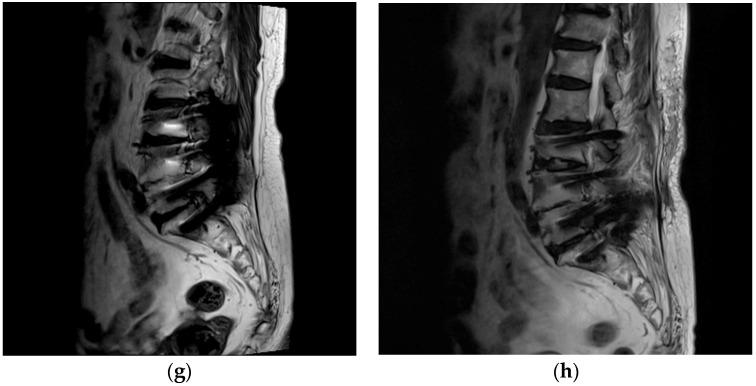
Preoperative and postoperative computed tomography and magnetic resonance images. (a) Preoperative coronal CT image showed that the effect of L5 pedicular kinking may be one of the reasons for the high tension of the L5 nerve root. (b) Postoperative coronal CT image showed partial pedicle excision to ensure adequate shoulder decompression of the L5 root. (c) Preoperative sagittal CT showed L5-S1 intervertebral foramen stenosis attributed to compression between the S1 superior articular process and the posterolateral osteophytes from the L5 vertebral endplate. (d) Postoperative sagittal CT showed enlarged L5-S1 intervertebral foramen after excision of the S1 superior articular process tip. (e) Preoperative axial CT showed the stenosis of the entrance zone of the left L5-S1 intervertebral foramen caused by the hyperplasia of the S1 superior articular process. (f) Postoperative axial CT showed enlarged L5-S1 intervertebral foramen by resecting the S1 superior articular process tip. (g) Preoperative MRI showed that the L5 nerve root was compressed at the L5-S1 intervertebral foramen. (h) Postoperative MRI showed the enlarged intervertebral foramen and the edema but uncompressed L5 nerve root.
